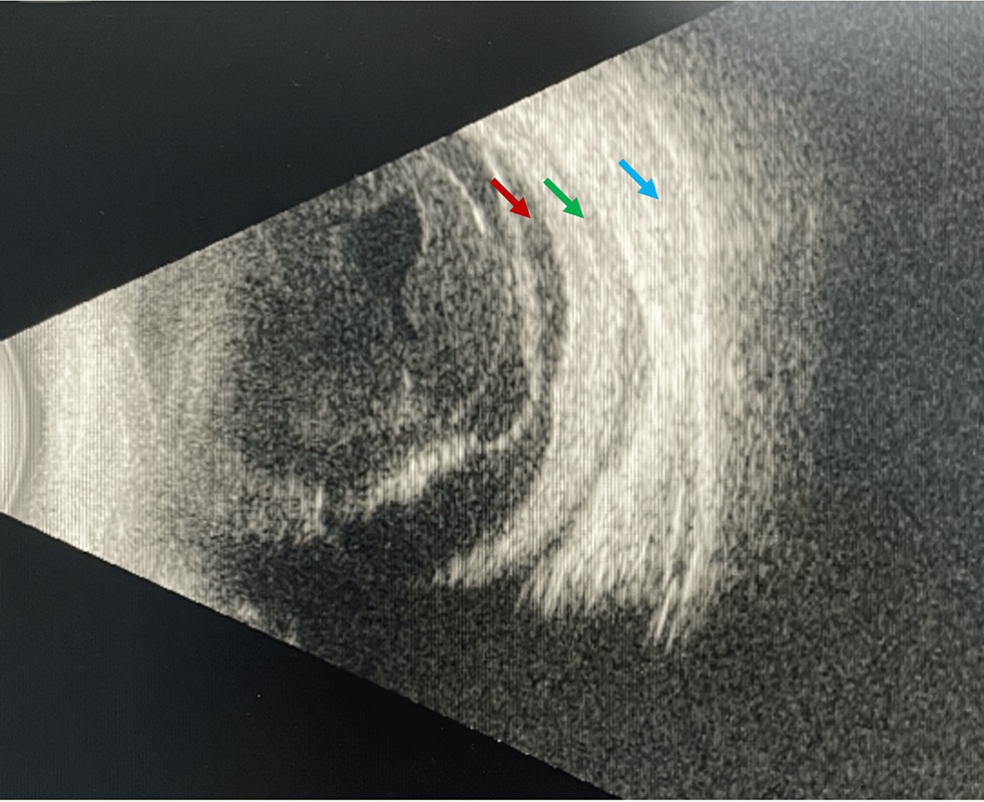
B-scan ultrasonography of the right globe showed retinal detachment (red arrow), thickened and detached choroid (green arrow), and thickened sclera (cyan arrow).
